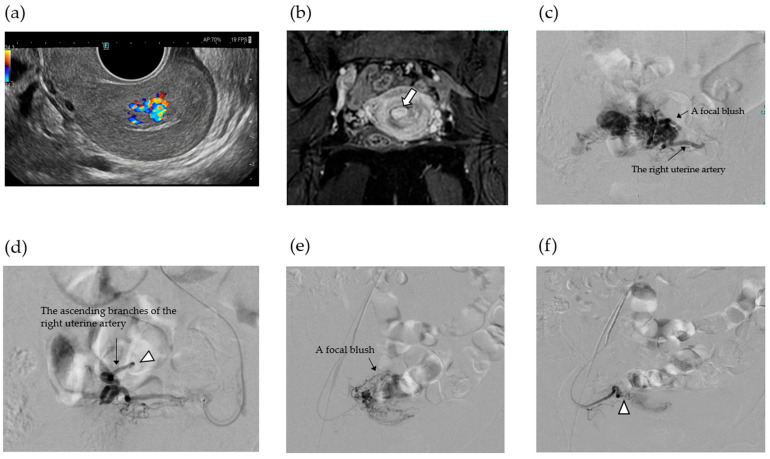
Transvaginal ultrasonography showing a hypervascular intrauterine mass (a). Sagittal contrast T2WI still demonstrated strongly contrasted retained products of conception (arrow) (b). Pelvic aortography showing a focal blush from the ascending branch of the left uterine artery (c). Post-embolization aortography showing contrast stasis without focal blush following complete embolization at the ascending branch of the left uterine artery using a gelatin sponge (arrowhead) (d). Pelvic aortography showing a focal blush from the ascending branch of the left uterine artery (c). Post-embolization aortography showing contrast stasis without focal blush following complete embolization at the ascending approximately 4 cm branch of the left uterine artery using a gelatin sponge (arrowhead) (d). Pelvic aortography shows a focal blush from the ascending branch of the right uterine artery (e). Postembolization aortography showing contrast stasis without a focal blush following complete embolization of the ascending branch of the right uterine artery using a gelatin sponge (arrowhead) (f).
